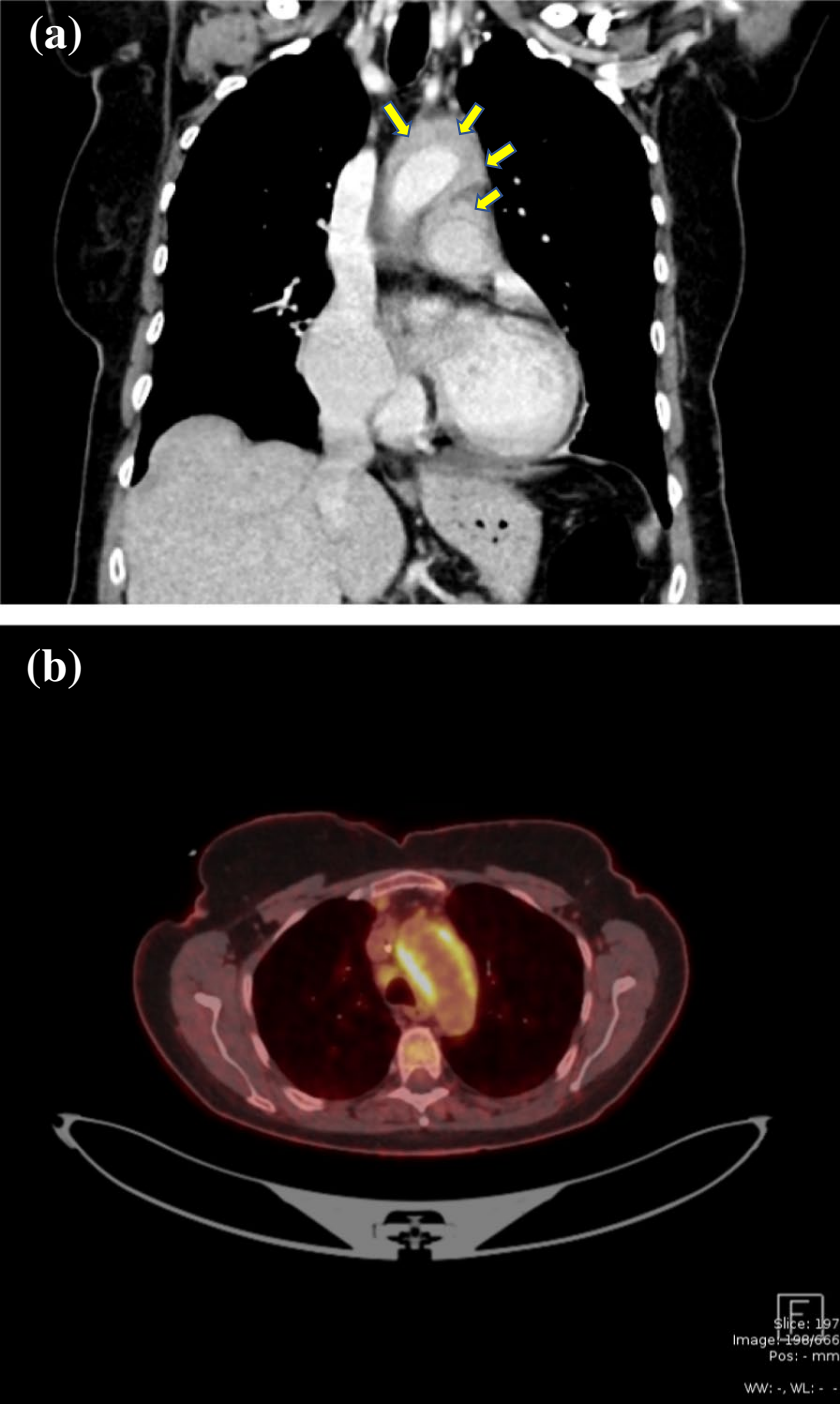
CT scan showing a markedly thickened aortic wall at the level of the aortic arch (1 cm) involving the wall of the pulmonary artery (a). PET scan showing the inflammation of the aortic arch (b)
